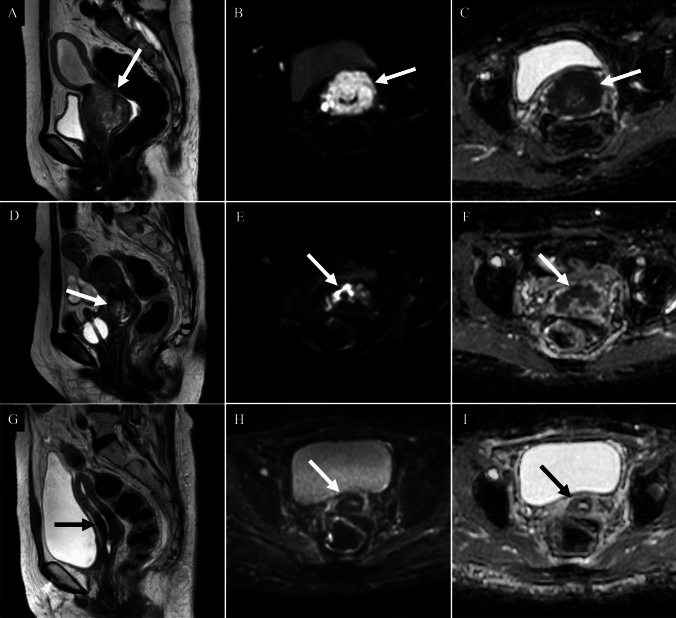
Squamous cell carcinoma of the uterine cervix in a woman in her 60 s treated with concurrent chemoradiotherapy. A, D, G Sagittal T2-weighted images; B, E, H Axial diffusion-weighted images; C, F, I Axial apparent diffusion coefficient (ADC) maps. A–C (before treatment): A bulky cervical mass with high signal intensity relative to the surrounding stroma is demonstrated on T2-weighted imaging (A: arrow), with marked diffusion restriction (B, C: arrows). The ADC was markedly reduced, with a mean value of 0.655 × 10⁻^3^ mm^2^/s. D–F (after 30 Gy pelvic irradiation): The tumor shows interval shrinkage on sagittal T2-weighted imaging (D: arrow). Diffusion-weighted imaging reveals residual high signal intensity in the right anterior cervical wall (E: arrow), corresponding to an area with an increased ADC of 1.018 × 10⁻^3^ mm^2^/s on the ADC map (F: arrow). G–I (a month after completion of all treatment, including intracavitary brachytherapy): Further regression is noted on sagittal T2-weighted imaging (G: arrow), with disappearance of the previously seen high signal on diffusion-weighted imaging (H: arrow). The ADC map (I: arrow) demonstrates a further increase in diffusivity, with a mean ADC of 1.436 × 10⁻^3^ mm^2^/s, consistent with complete response and reconstitution of the low-signal stromal ring
